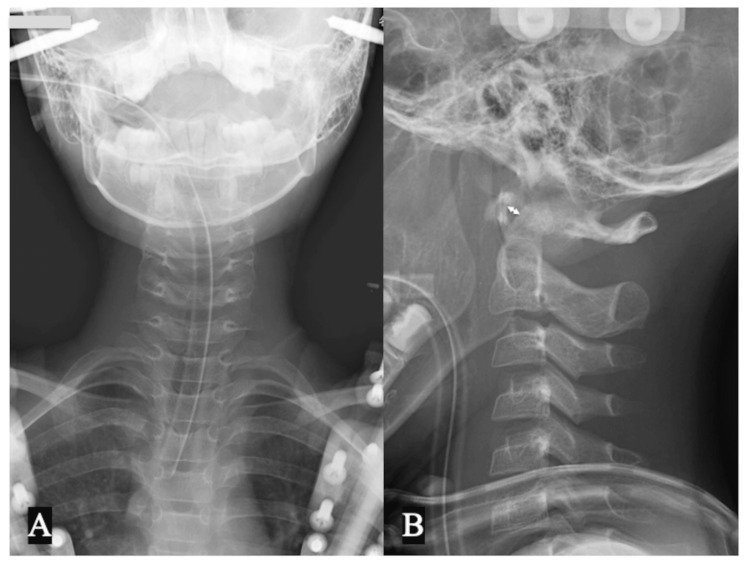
Cervical spine X-rays after halo vest application Plain radiograph of the cervical spine immediately after halo vest application showing a reduction in (A) the torticollis and (B) atlantoaxial subluxation; atlantodental interval 3 mm (double-headed arrow)
